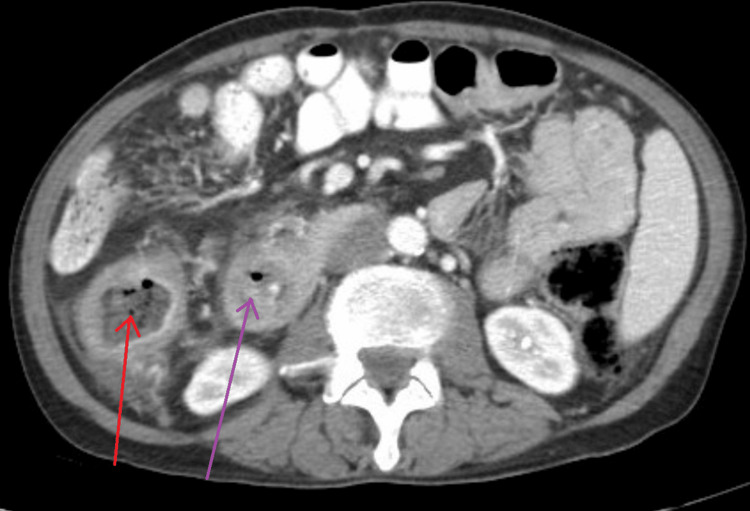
Abdominal CT demonstrating circumferential mural thickening of the ascending colon (red arrow) and duodenum (purple arrow)
